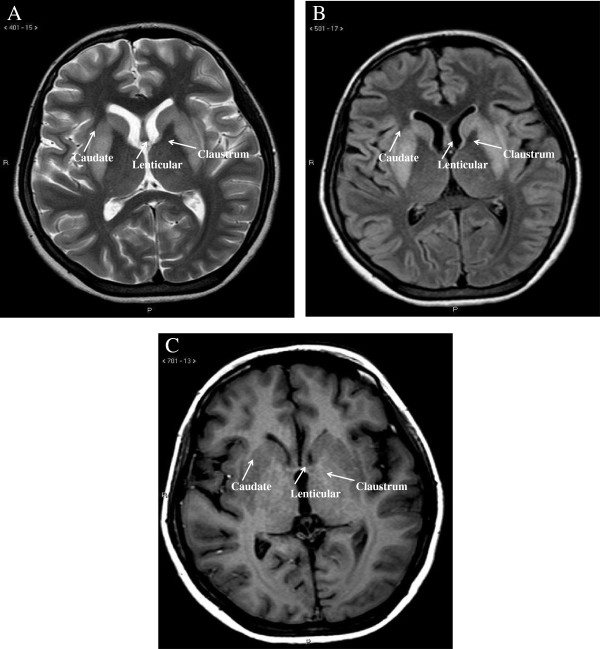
MRI features. Signal intensity of the lenticular, claustrum, and caudate cerebral nuclei on axial T2 weighted images (A), FLAIR images (B) and Tl weighted images (C).
